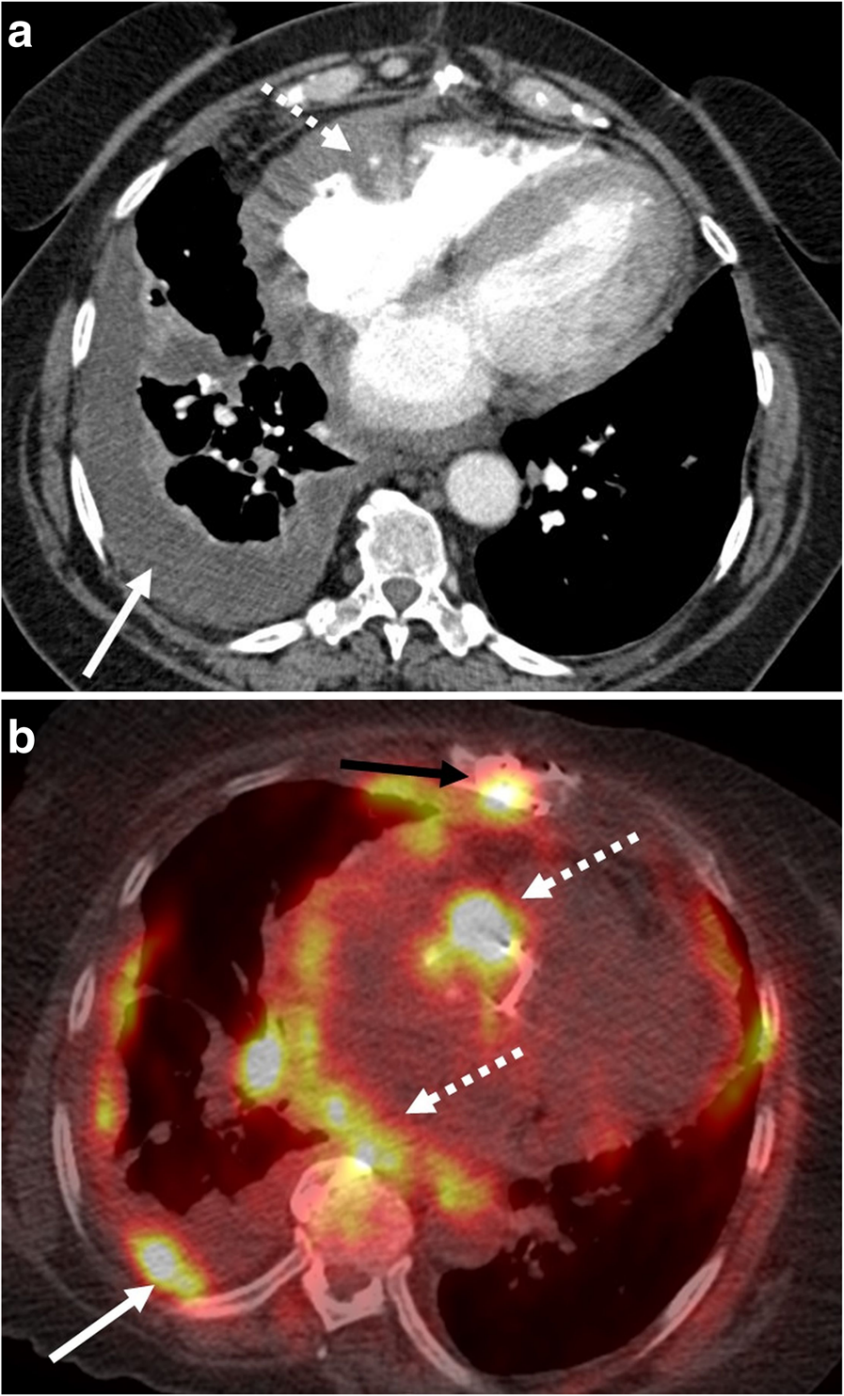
Pleural and pericardial involvement. A 62-year-old female presenting with chest pain and shortness of breath. a Contrast-enhanced axial CT image demonstrates a partially loculated right pleural effusion (solid arrow) and mass-like thickening of the pericardium extending into the epicardial fat encasing the right coronary artery (dashed arrow). b Fused axial PET-CT demonstrates associated hypermetabolic uptake within the pericardial mass (white dashed arrows) and better depicts multifocal right-sided pleural involvement (white solid arrow). There is also sternal involvement (black solid arrow)
